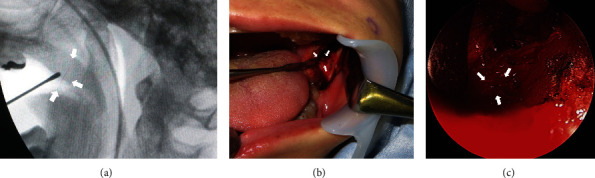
(a) Intraoperative X-ray transmission device images. The position of the broken injection needle was confirmed with a sonde using fluoroscopy. (b) Intraoperative photograph. (c) Endoscopic image taken intraoperatively. An incision approximately 25 mm in length was made on the oblique line of the mandible. A broken injection needle pointing upward from the base of the coronoid process was confirmed in the medial pterygoid muscle. Broken injection needles are shown by an arrow.
